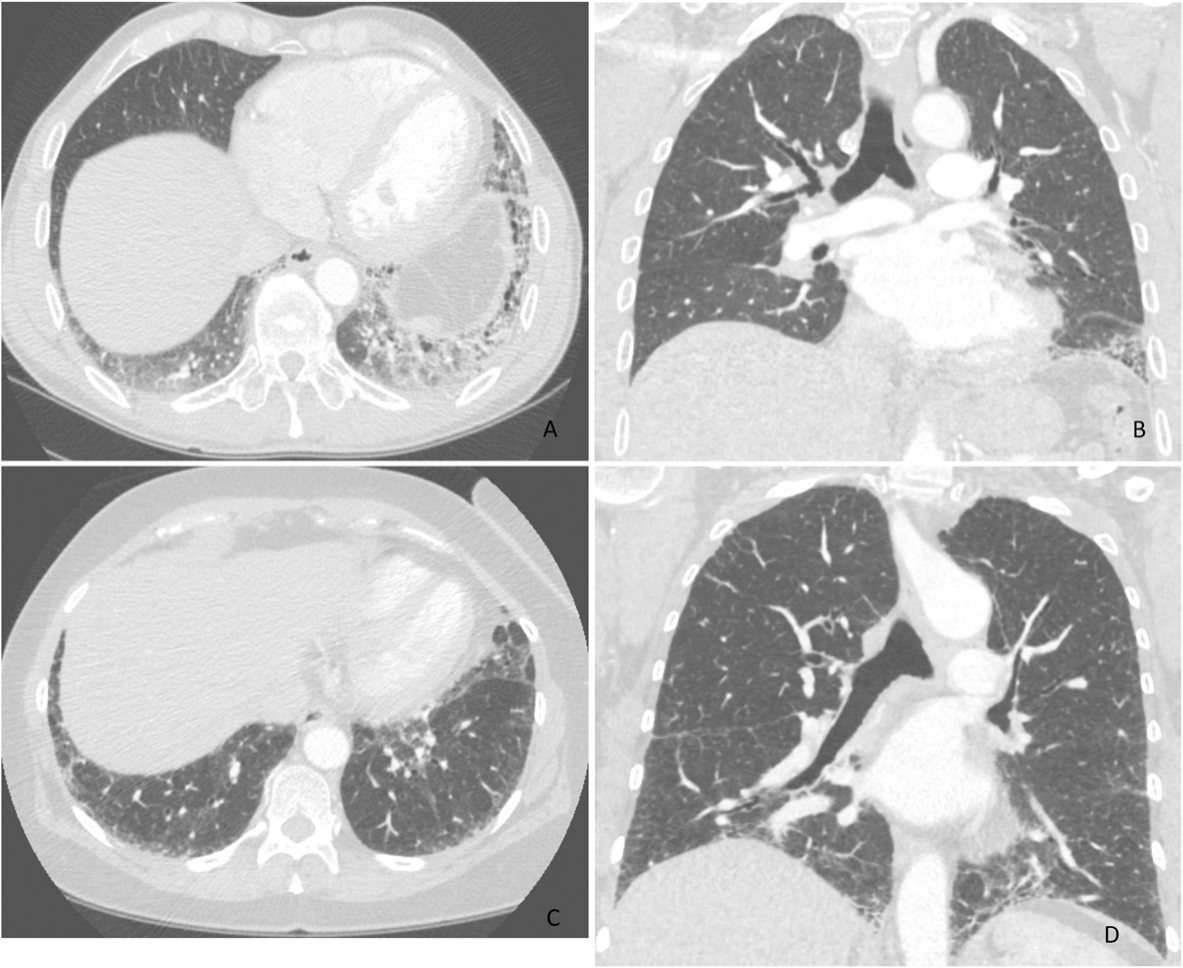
High resolution computed tomography (HRCT) scans of two patients with the *TERC* variant. A-B: HRCT scan of patient number 1 shows a left lung predominant usual interstitial pneumonia pattern with basal and peripheral distribution, reticular abnormalities, traction bronchiectasis and honeycombing. C-D: HRCT scan of patient number 2 reveals fine reticular abnormalities and traction bronchiectasis without honeycombing with basal and peripheral distribution
